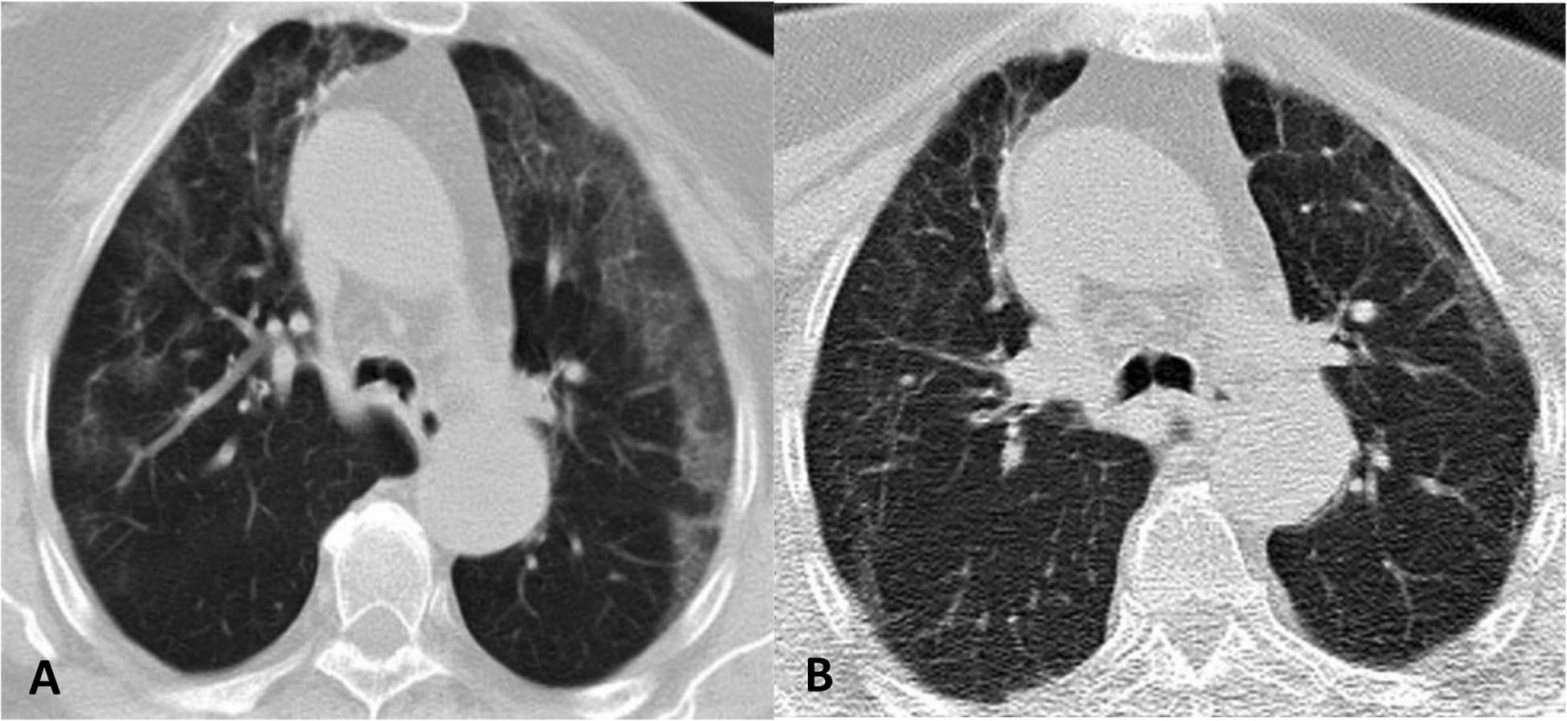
A 55-year-old RT-PCR (+) female patient with fever, cough, and abdominal pain. a Initial CT image obtained shows bilateral peripherally located ground glass areas. b 2nd month follow-up CT image shows almost complete regression of ground glass opacities and shows newly developed fibrous stripes
